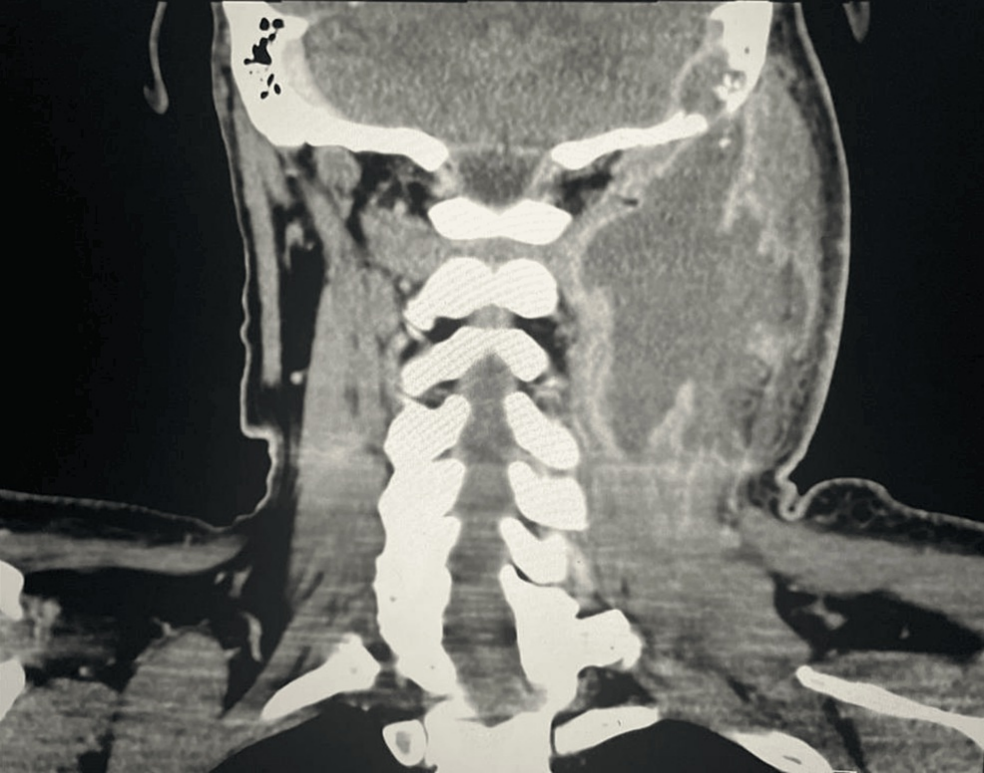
Non-contrast coronal CT scan of the neck revealing well-defined BA measuring 10 cm in diameter BA: Bezold's abscess
